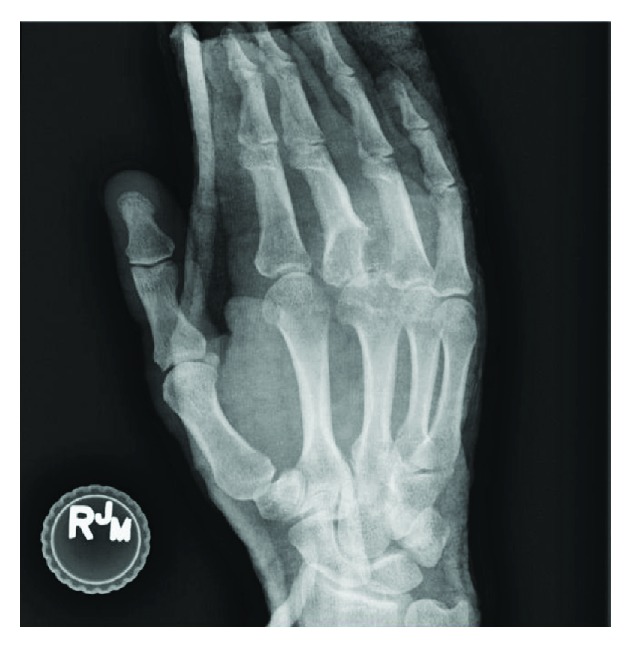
Oblique radiograph of the right hand demonstrating a lytic destructive process of the base of the proximal phalanx, third digit.
